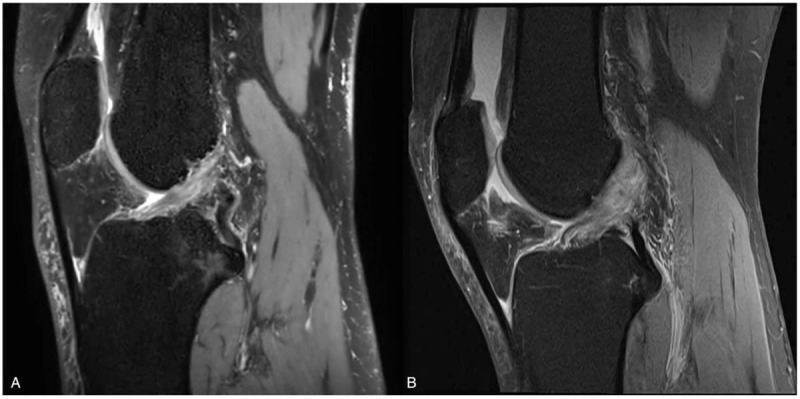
MRI knee Rt.(non-enhance sagittal view): comparison with follow up MRI (A) and immediately after injury MRI (B). MRI = magnetic resonance imaging.
